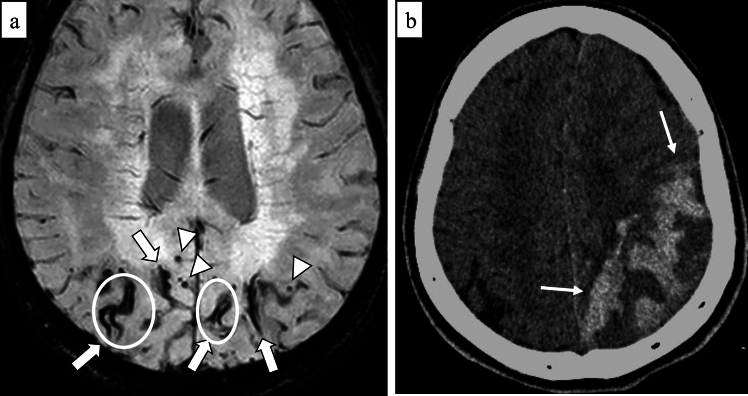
A 79-year-old male with probable CAA. MMSE score is 18. SWI (a) shows cSS (arrows) with track-like appearance (circles), and also lobar CMBs (arrowheads) in bilateral occipital lobes. One year later, CT (b) shows a hemorrhage with finger-like projections (small arrows) in the left parietal lobe
